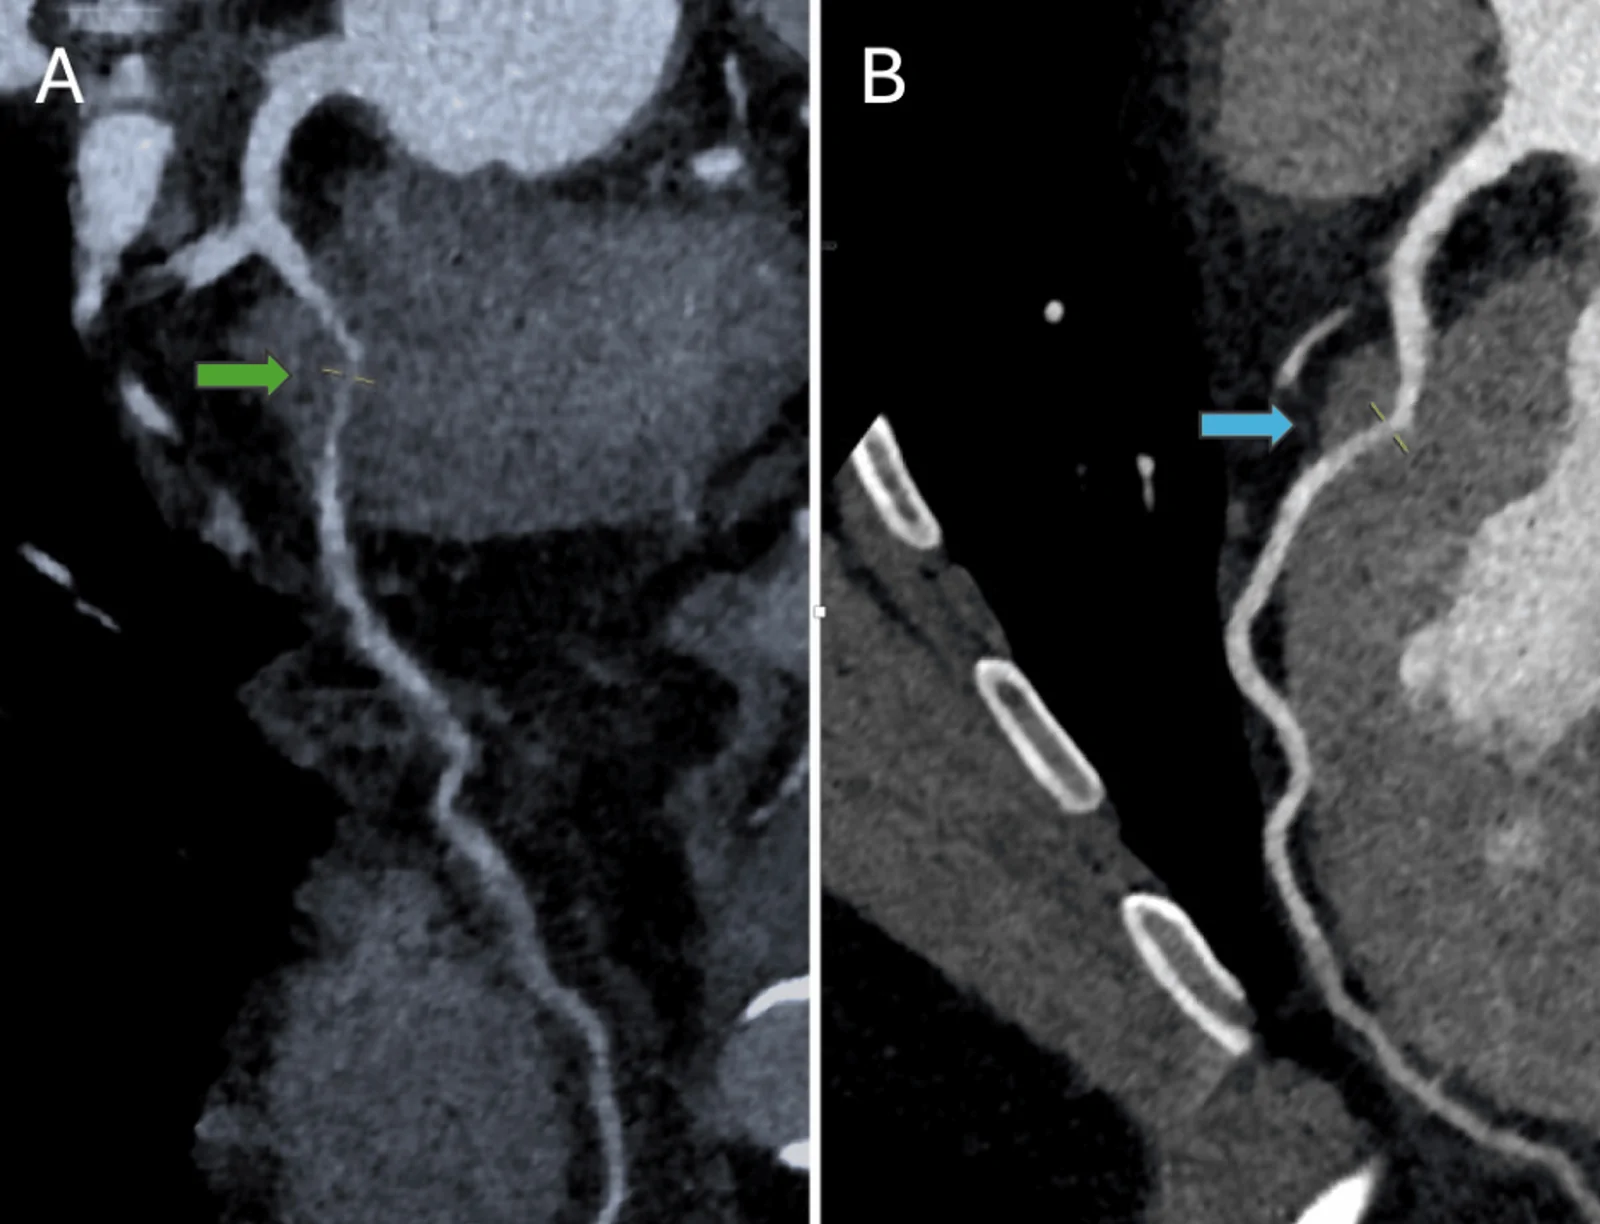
Coronary computed tomography angiography images Curved planar reformation technique revealing a 20 mm long and 7 mm deep myocardial bridge of the mid-LAD, with luminal obstruction of at least 75% during systole (A, green arrow) and adequate filling during diastole (B, light blue arrow). LAD: left anterior descending coronary artery
